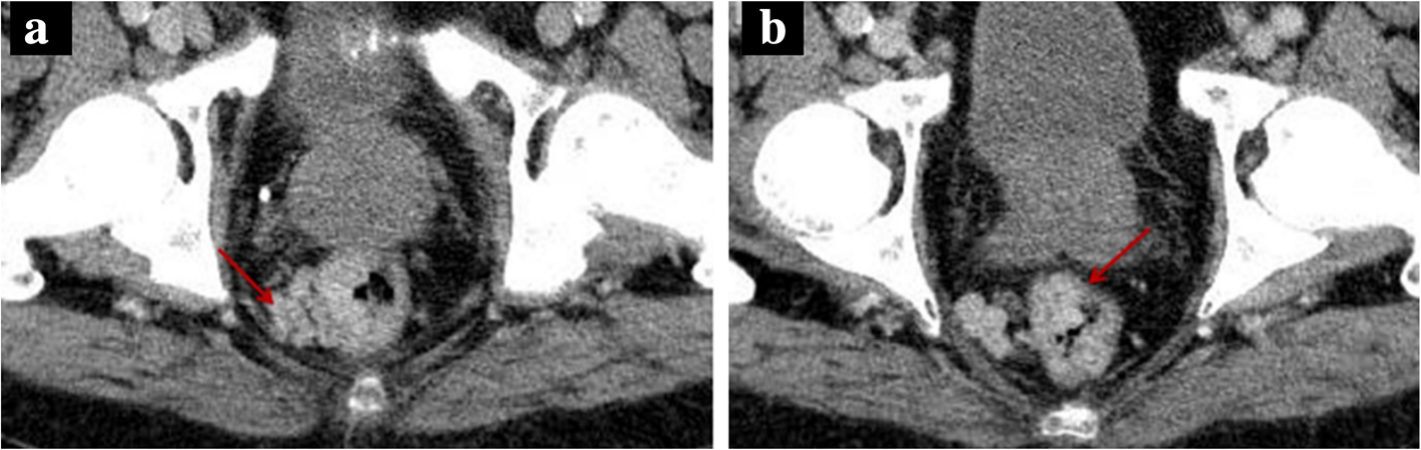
Anorectal/hemorrhoidal varices. Portal venous phase CT images showing a variceal network along, around and within the anorectal wall. In this case, we can individualize ingurgitated tortuous vessels (red arrows), but sometimes thickening and hyperenhancement are all we can see
